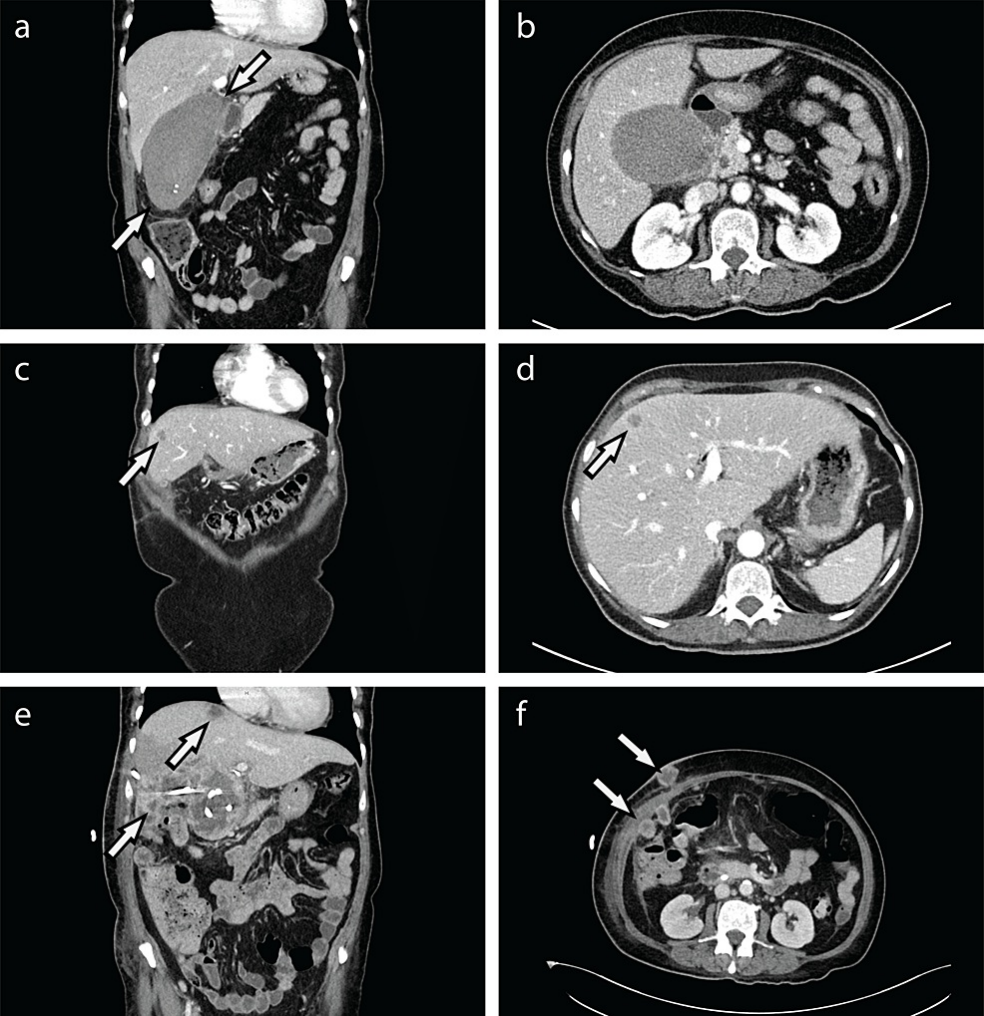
CT images upon initial admission and post-drainage (a/b) Coronal and axial CT imaging with contrast of the abdomen upon initial admission to ED. The length of the gallbladder measured along its greatest length was approximately 13 cm (between the white arrows). (c/d) Coronal and axial CT imaging with contrast of the abdomen upon readmission to ED. There are multiple new hypoattenuating lesions in the liver (white arrows). No lesions of the omentum or abdominal wall are noted. (e/f) Repeat CT imaging following three rounds of CT-guided drainage. There are now multiple lesions involving the liver, omentum, and anterior abdominal wall containing internal fluid components with peripherally thick, irregular, enhancing walls.
